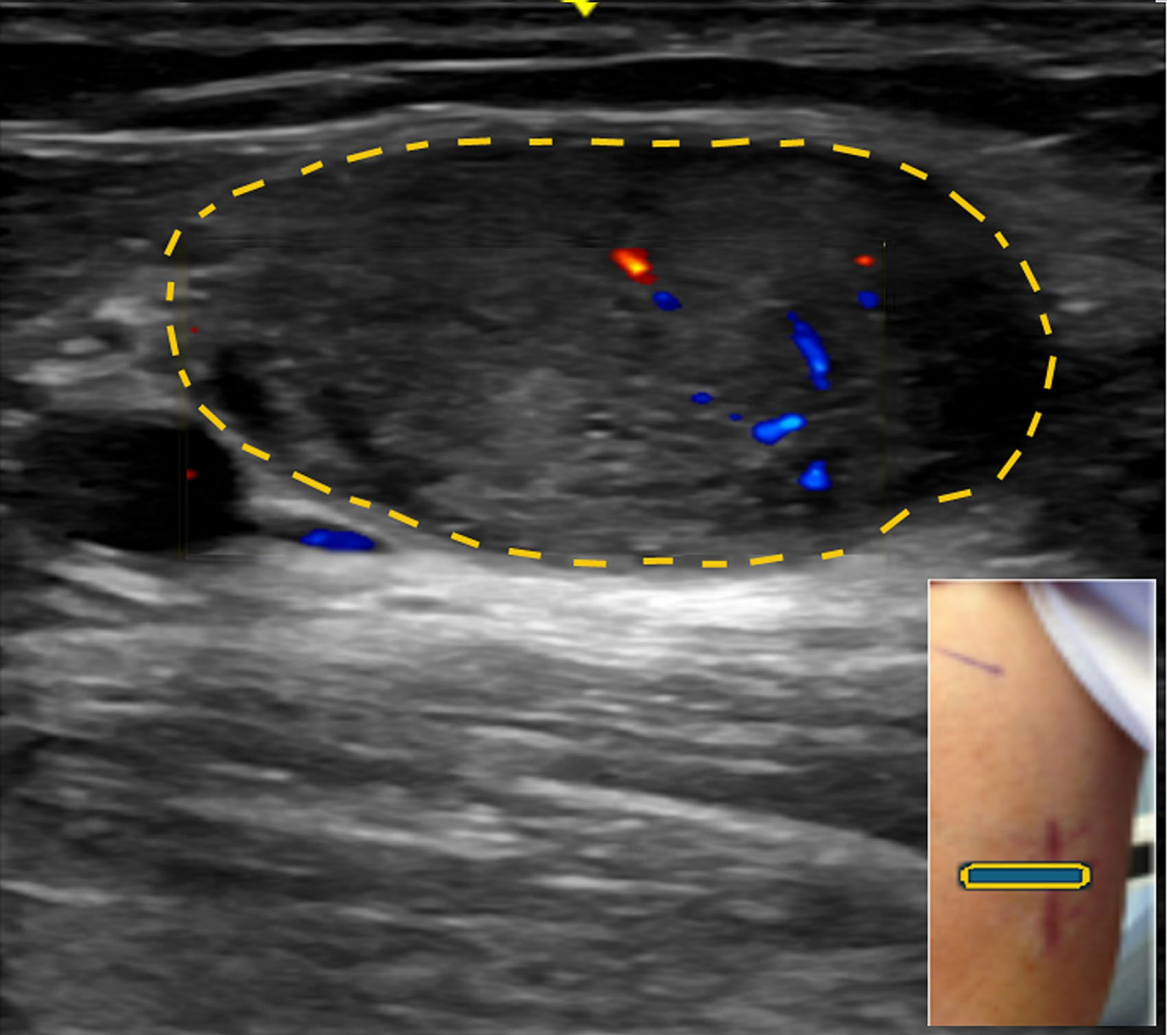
Short axis view with color Doppler at the mid-upper arm showing a schwannoma in the median nerve.
